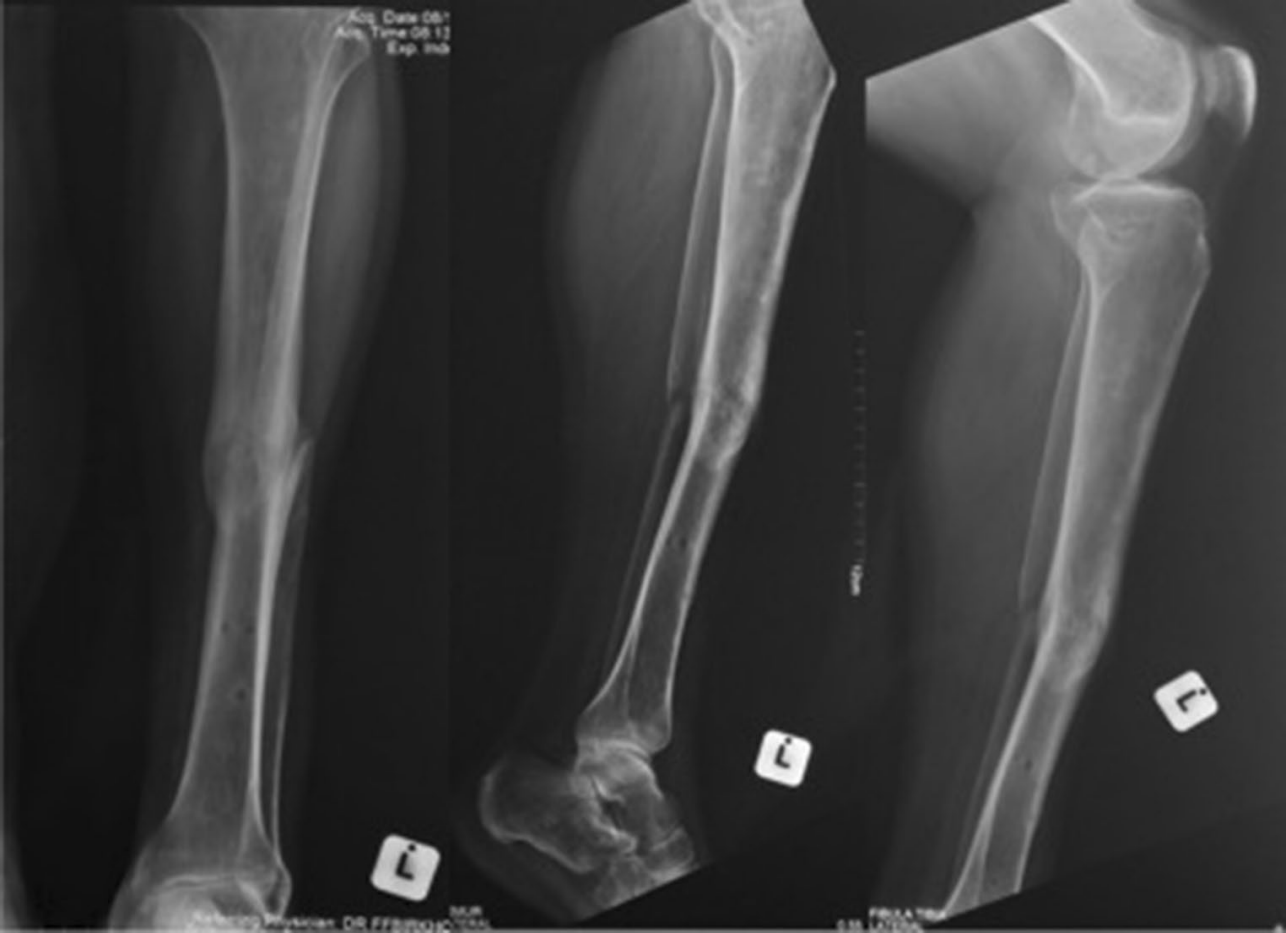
Radiograph series of 2-year follow-up. Fracture united and bone is aligned
